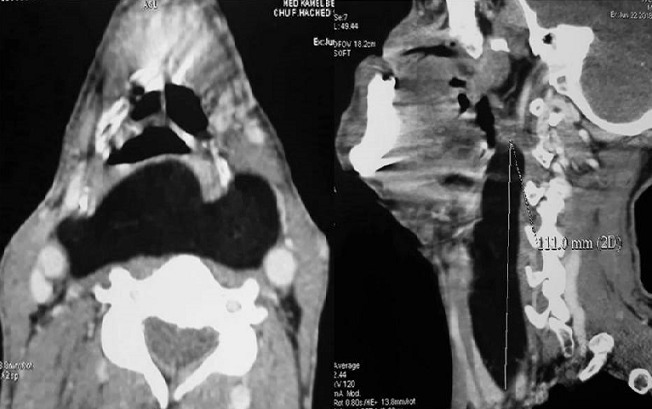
CT (computed tomography) image of the head and neck shows a homogeneous, low density mass measuring 110 cm × 73 cm × 26 cm in the posterior pharyngeal wall, extending from C2 to C7
